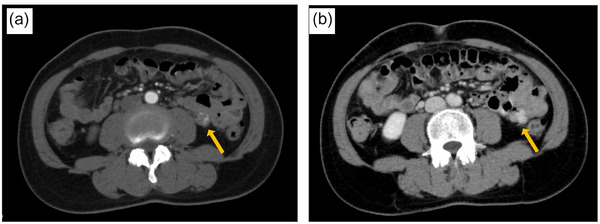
Contrast‐enhanced computed tomography (CT). (a) Arterial phase of contrast‐enhanced computed tomography showed contrast leakage into the lumen of the small intestine. (b) The late phase showed an increase in leaked contrast.
